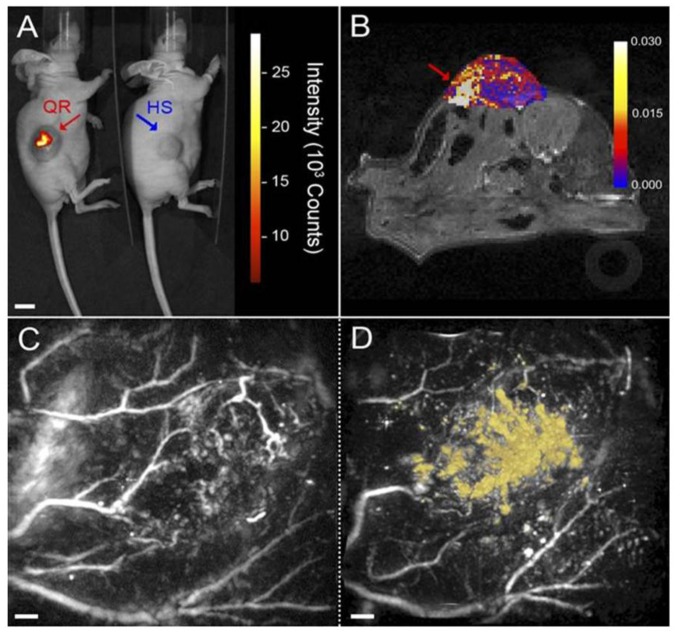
Multimodal *in vivo* imaging of quantum rattles (QRs): (A) NIR fluorescent intensity in the areas where QRs (red) and non-fluorescent hollow mesoporous silica shells (HS) control (blue); (B) MR image obtained following the injection of QRs; and 3D photoacoustic images of tumors acquired at 670 nm before (C) and after (D) the injection of QRs. Reproduced with permission from [191]. Copyright Proceedings of the National Academy of Sciences of the United States of America, 2015.
